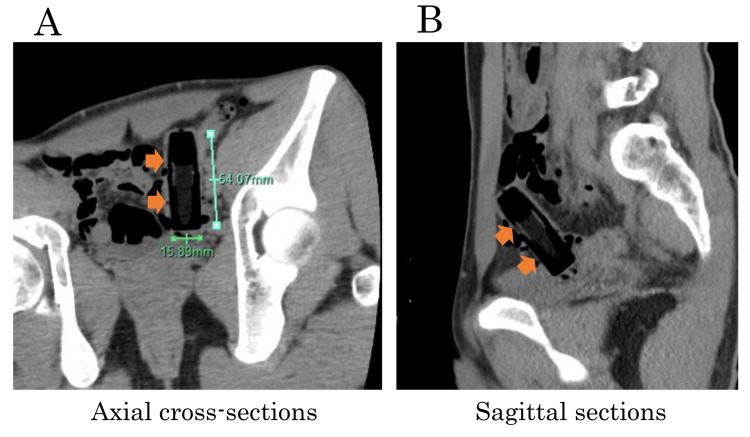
CT findings Abdominopelvic CT shows no evidence of gastrointestinal disturbance, as there is no free air, ascites, or inflammation around the intestinal tract. Axial (A) and sagittal (B) sections of the CT are shown. A rod-shaped foreign body (orange arrow) has strayed into the upper rectum.
